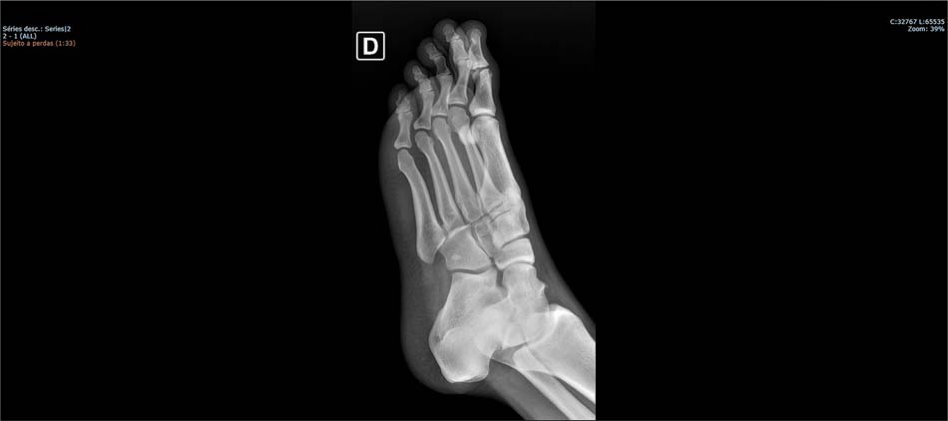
Preoperative X-ray of the foot in lateral view
